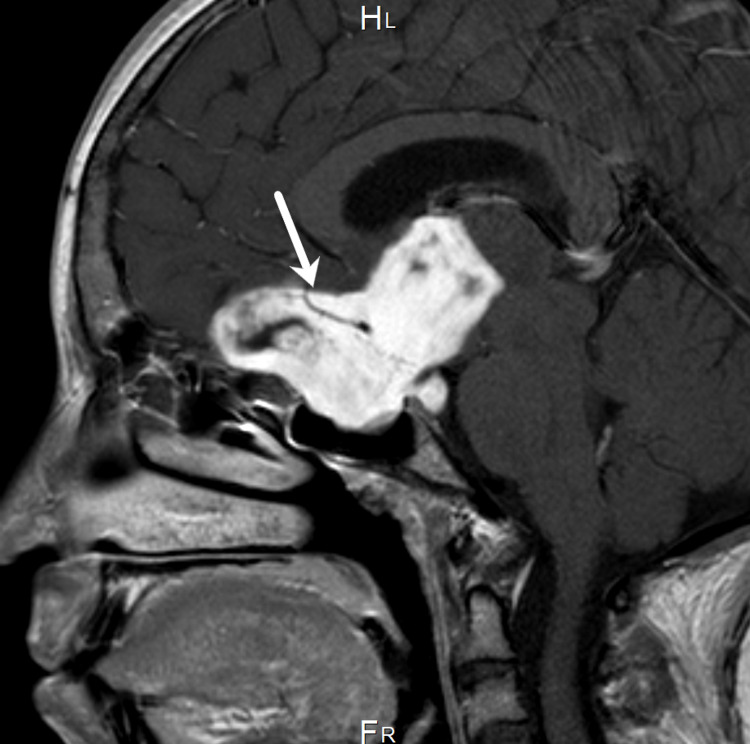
T1-weighted brain MRI post-contrast sagittal view shows the large sellar/supraseller mass engulfing the anterior portion of the circle of the Willis with extension to the third ventricle.
